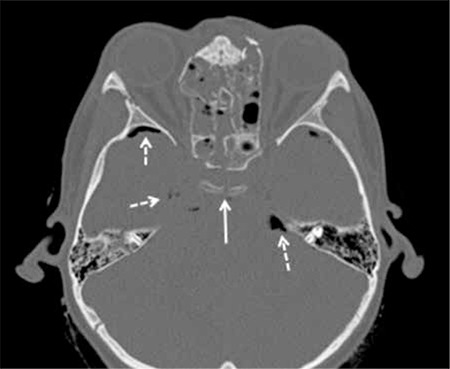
Axial unenhanced computed tomography image at the level of the orbits shows displaced fractures through the nasal bridge, ethmoid sinus walls, nondisplaced fracture through the right lateral orbital wall and depicts a fracture extension through the skull base and sella (arrow). Also, mix type fracture of the right temporal bone, loss of aeration of the right mastoid air cells and foci of pneumocephalus (dashed arrow) are observed
